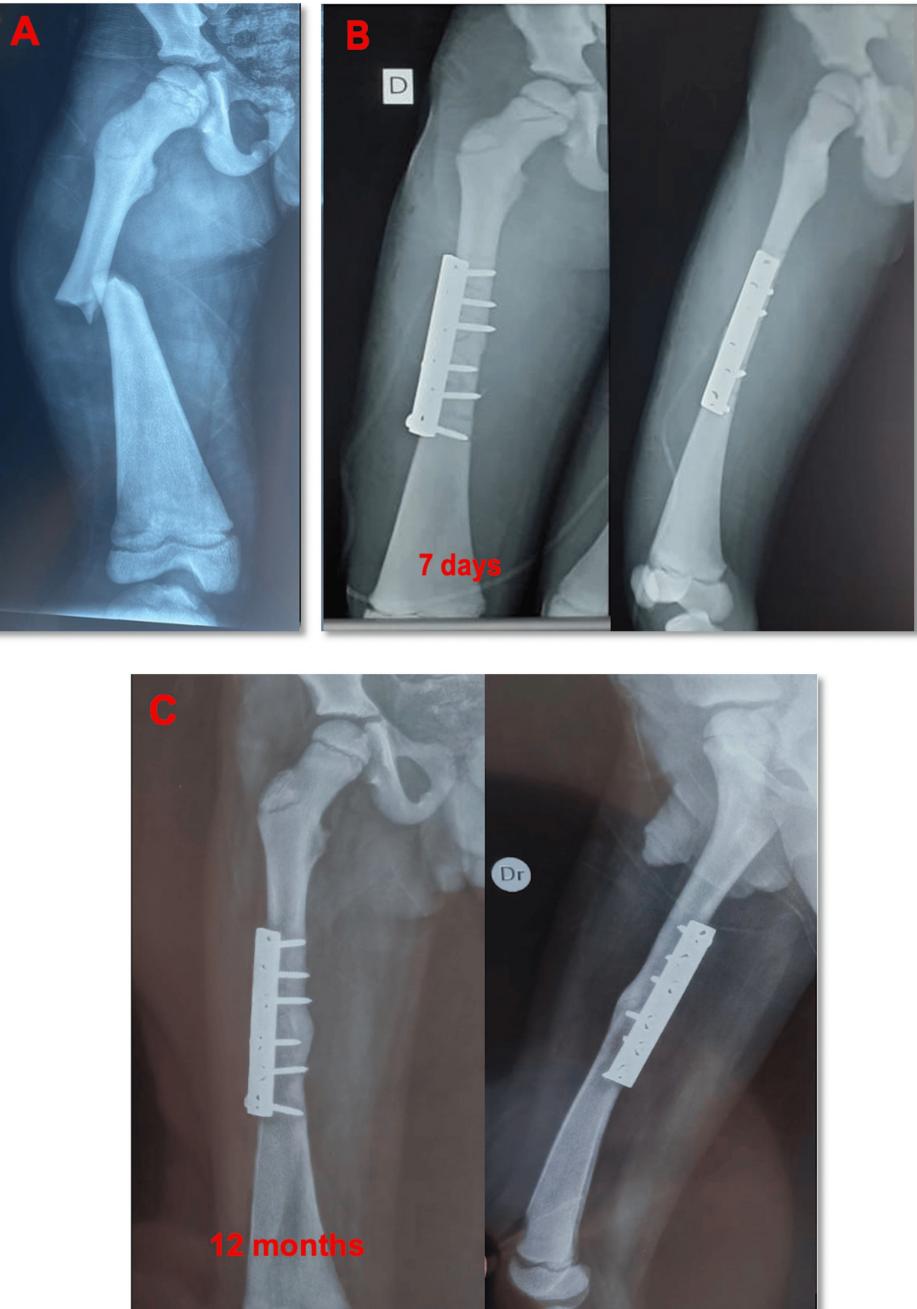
(A) A displaced transverse fracture of the right femoral diaphysis with a narrow medullary canal, (B) postoperative control radiographs after seven days, and (C) control radiographs after 12 months
